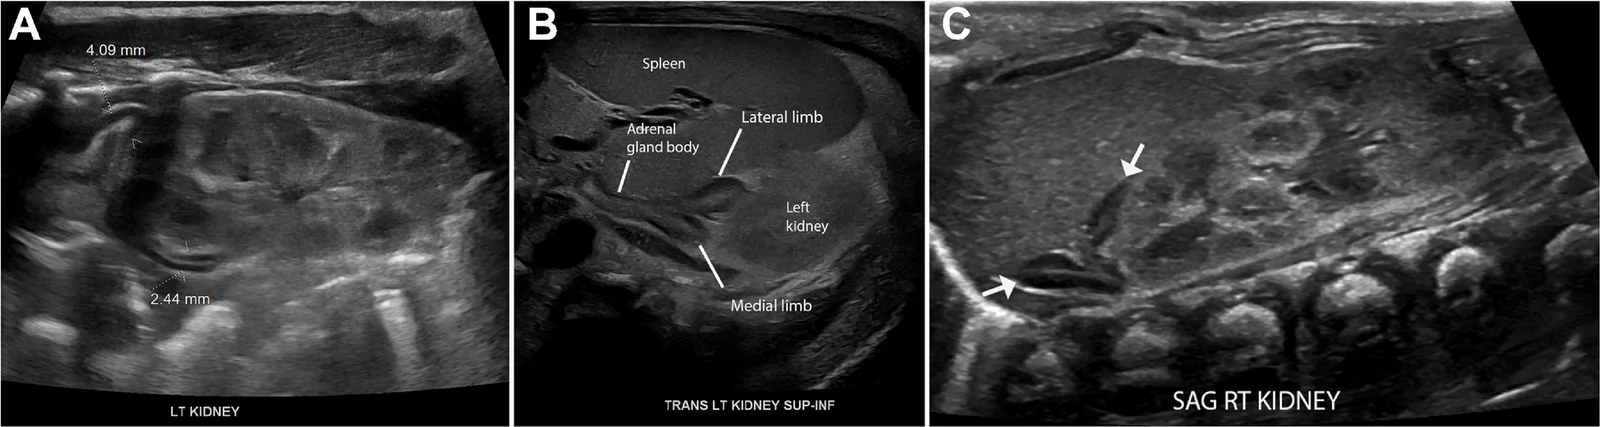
Normal adrenal glands in a 1-day-old newborn male infant imaged by ultrasound for renal function concerns, compared to normal adrenal glands in a 10-day-old infant’s renal ultrasound for antenatal diagnosis of urinary tract dilatation. a Longitudinal and (b) transverse views of the left adrenal gland and left kidney in a 1-day-old infant, showing normal architecture of the adrenal limbs, with hypoechoic cortex and thin echogenic stripe of the medulla. Calipers (a) denote thickness of each adrenal limb. c Longitudinal view of the right adrenal gland (*arrows*) and kidney in a 10-day-old male infant demonstrates a typical relative proportion of the adrenal gland length to renal length in infants
